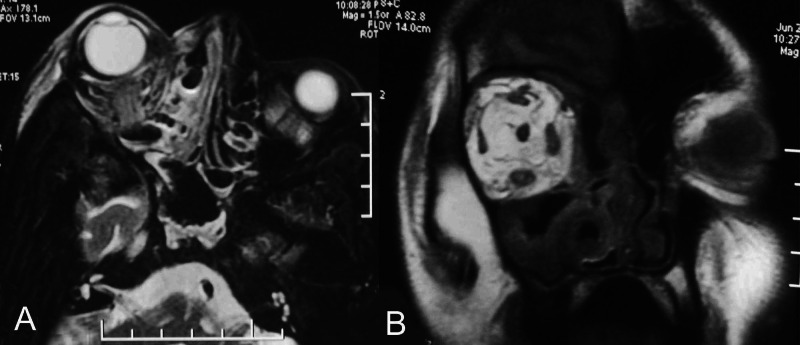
Magnetic resonance imaging of the orbit (A) Axial MRI (T2) showing hyperintensity of the ethmoid and nasal sinus and of the retrobulbar space (B) coronal section (T1) showing irregular hyperintensity of the retrobulbar space
